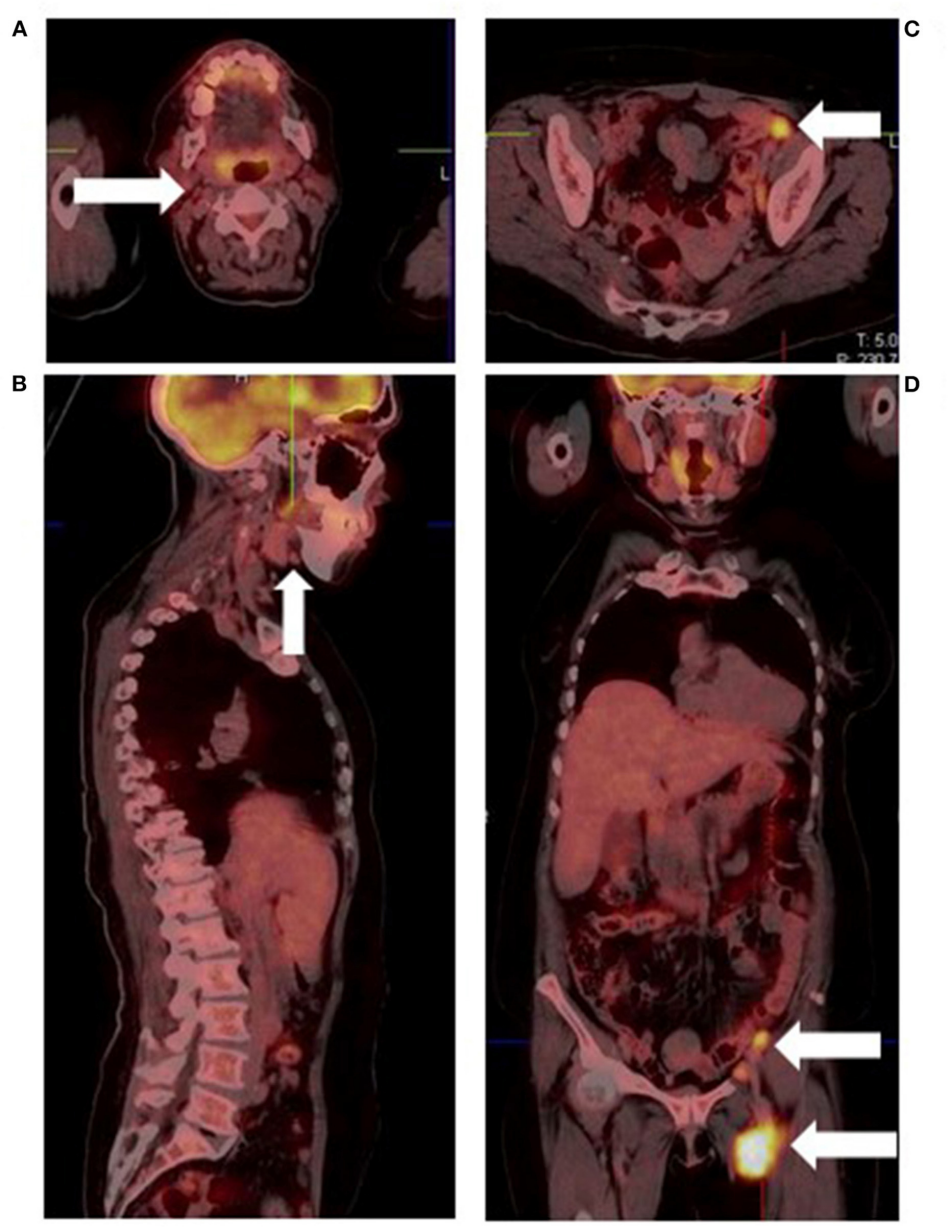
Malignant melanoma of the left heel in a known retroviral disease patient. Fused [18F]FDG PET/CT images show cervical (A), submandibular (B), and abdominopelvic lymphadenopathy (C,D). Based on their proximity to the primary site and intense hypermetabolism, the abdominopelvic lymph nodes represent metastatic sites. Conversely, the low grade cervical and submandibular lymph nodes most likely represent retroviral disease.
